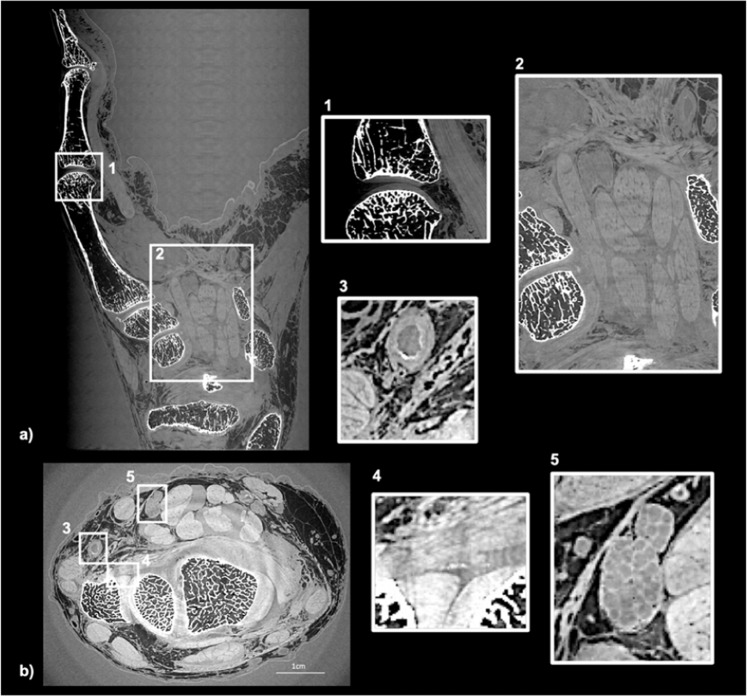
PCI-CT slices of a human hand and wrist. (a) Coronal section showing mainly the digitus minimus manus. (b) Axial slices in the wrist region. The insets present zoom in the (1) interphalengeal proximal joint, (2)the carpal tunnel, (3) an artery, (4) an enthesis and (5) the median nerve.
